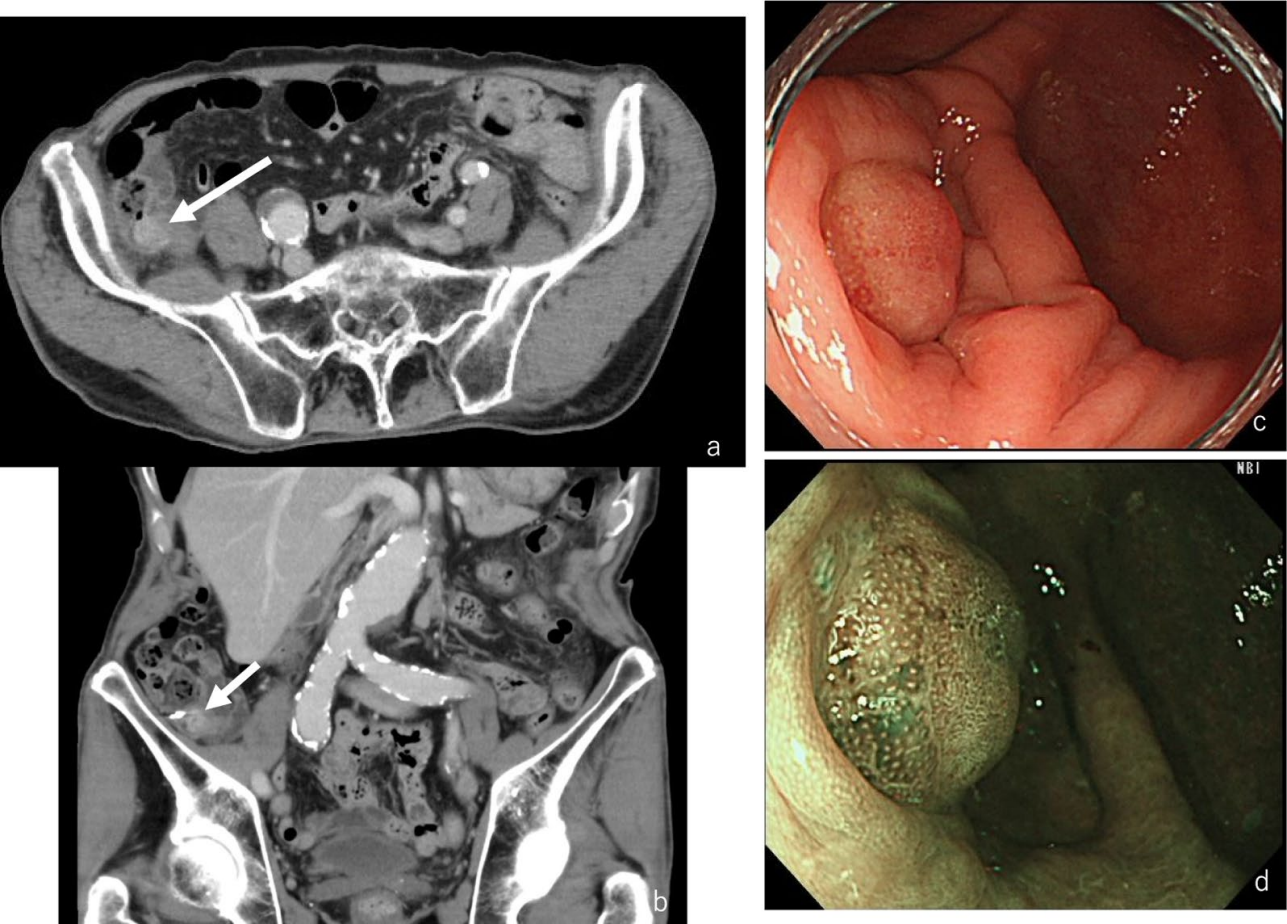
Image findings. a, b Contrast computed tomography scan findings. CT scan revealed a 15-mm nodular lesion with contrast effect at the appendiceal orifice. There was no metastatic lymph node swelling. c, d Colonoscopy findings. Colonoscopy showed a 10-mm elevated lesion at the appendiceal orifice
